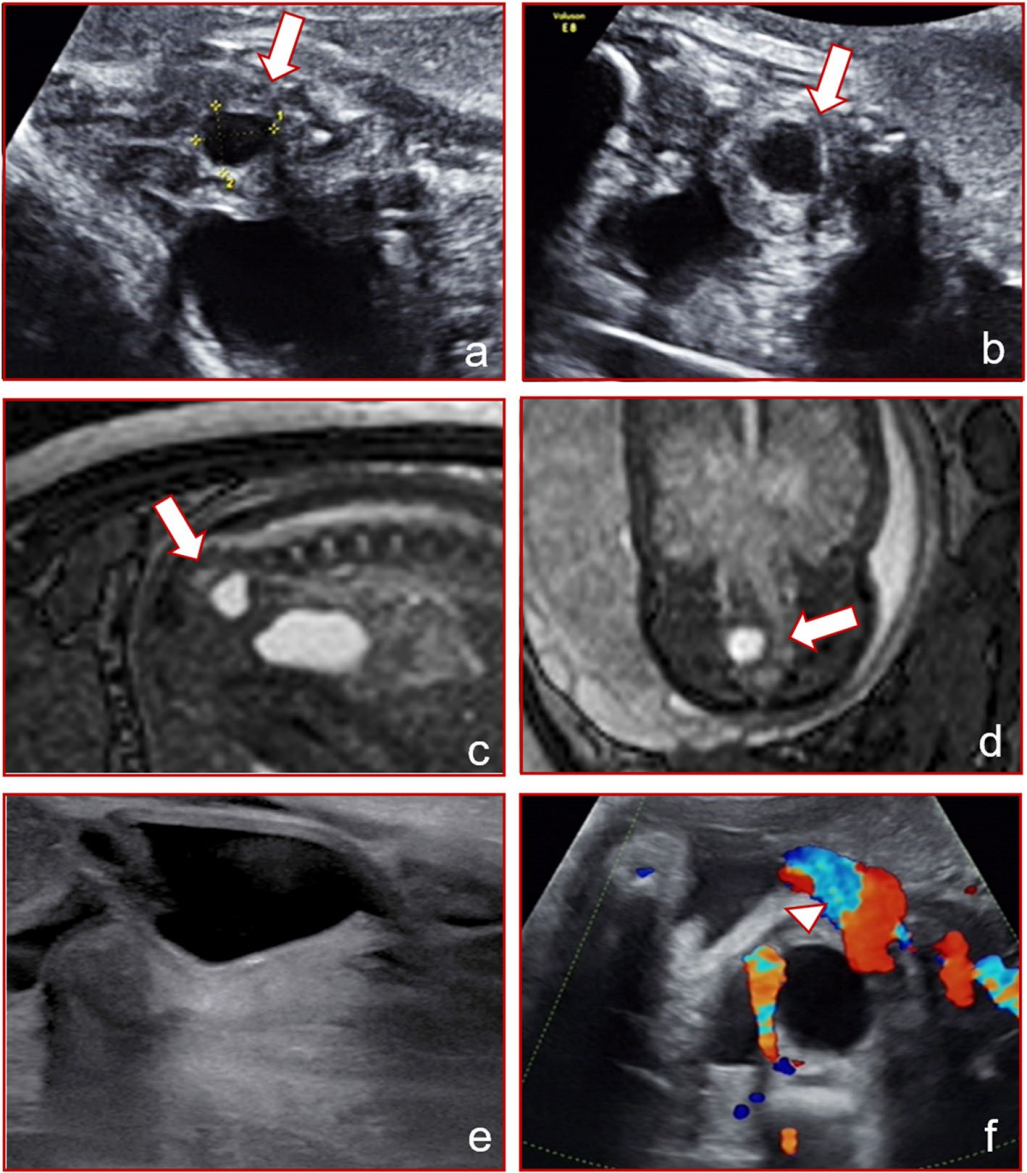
Comparison between the case of uterine effusion showing an anechoic cyst (*arrow*) and ovarian cyst (*arrowhead*): ultrasound imaging of uterine effusion in different sections at 28^+ 2^ weeks of gestation (a and b); MRI images of uterine effusion in different sections at 28^+ 2^ weeks of gestation (c and d); postnatal image of the uterus (e); ultrasound image in another case of ovarian cyst at 31 weeks of gestation for contrast (f), the diagnosis was confirmed by postnatal ultrasonography
